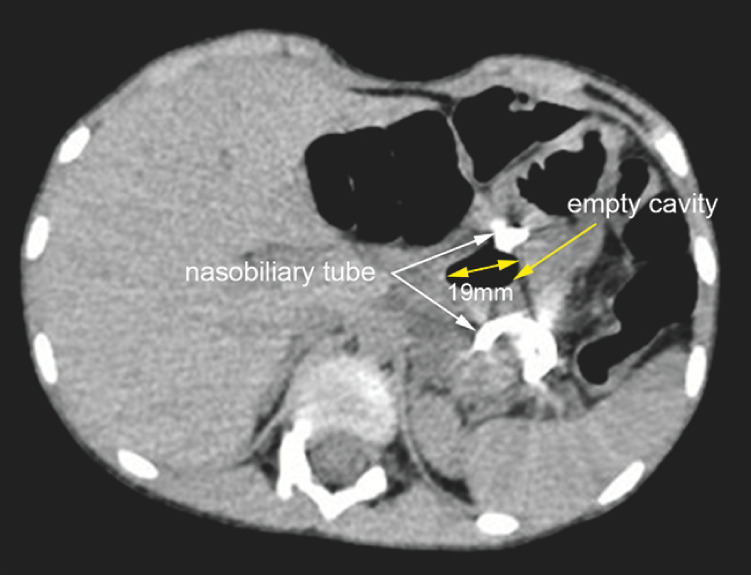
CT at 1 week after start of external nasobiliary drainage showed shrinking of the pseudocyst to 20.9 × 16.3 × 21.6 mm.
